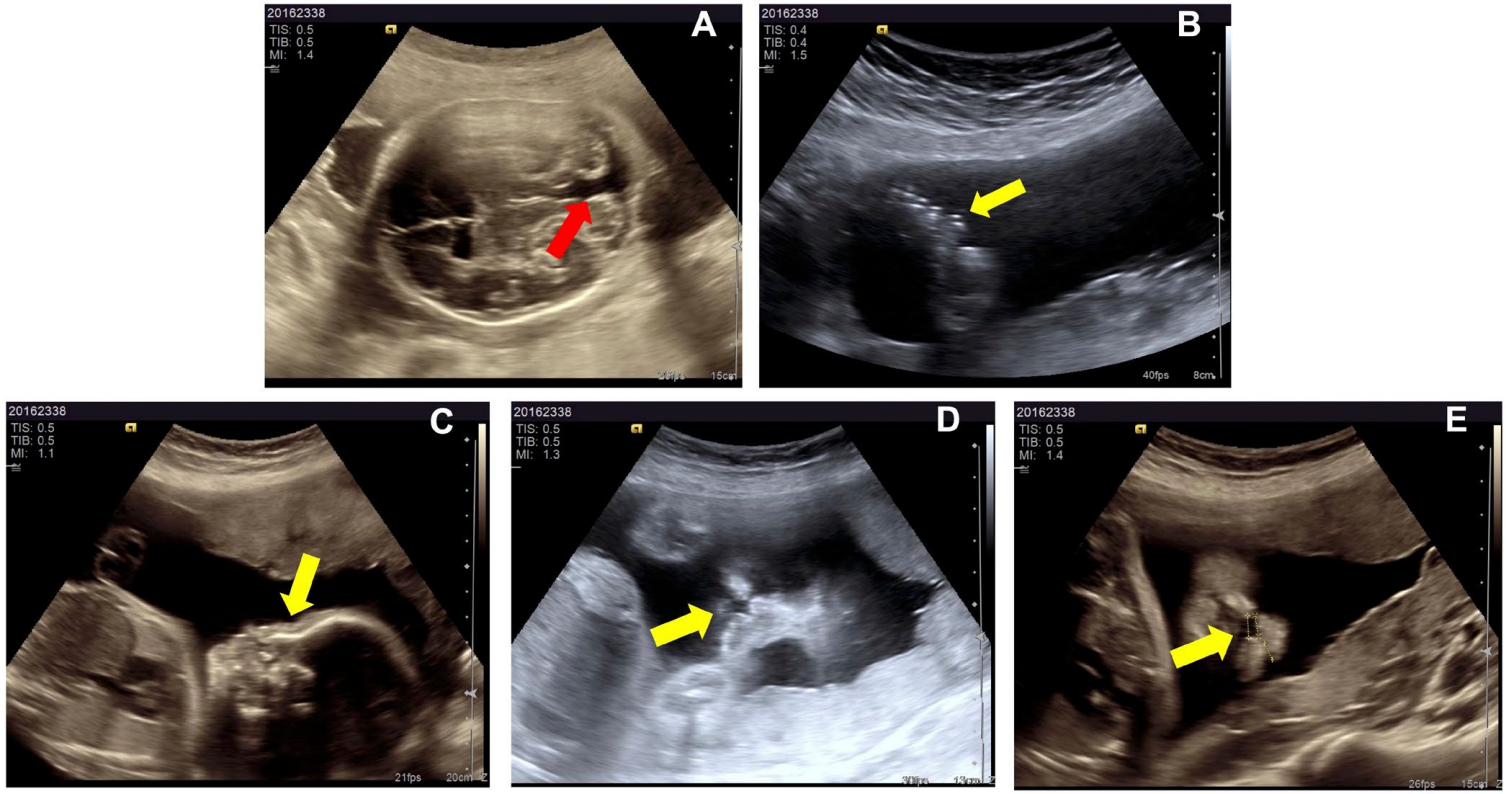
Ultrasonography examination of the fetus at 26 weeks of gestation from the second adverse pregnancy. (A) Absence of the cerebellar vermis, with continuity between the cerebellomedullary cistern and the fourth ventricle (red arrow). (B) Polydactyly (yellow arrow). (C) Flat nasal bridge (yellow arrow). (D) Cleft lip (yellow arrow). (E) A discontinuity of approximately 0.65 cm in the echogenic continuity of the upper lip, with a 0.3 cm discontinuity in the echogenicity of the alveolar arch on the right side (yellow arrow). Ultrasound images taken at 26 weeks of gestation.
